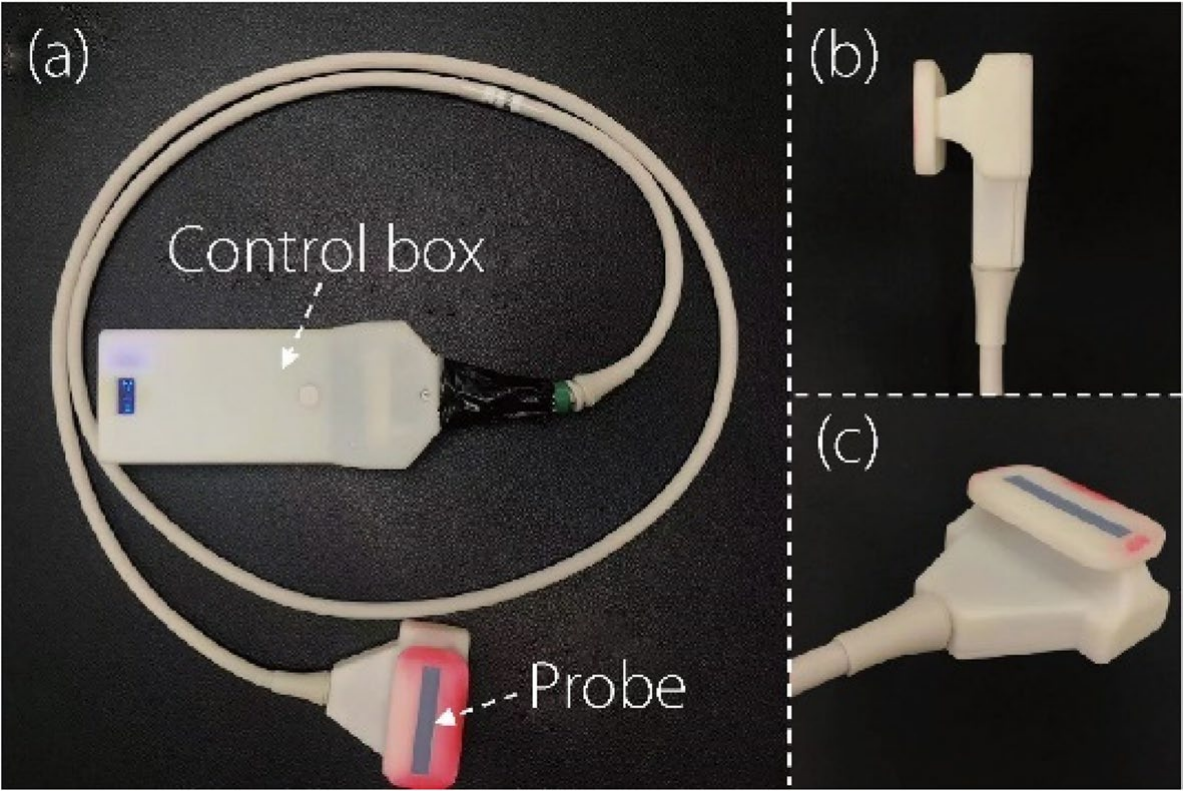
Wearable RUSI biofeedback system: ultrasound image acquisition unit wirelessly connected to the matched smartphone-based application
